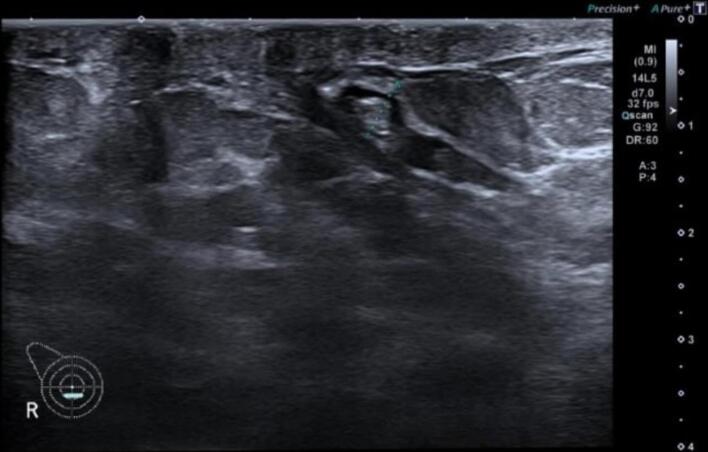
Ultrasound of right breast before treatment shows retro areolar dilated duct with internal avascular echogenic material at 6’o clock.
